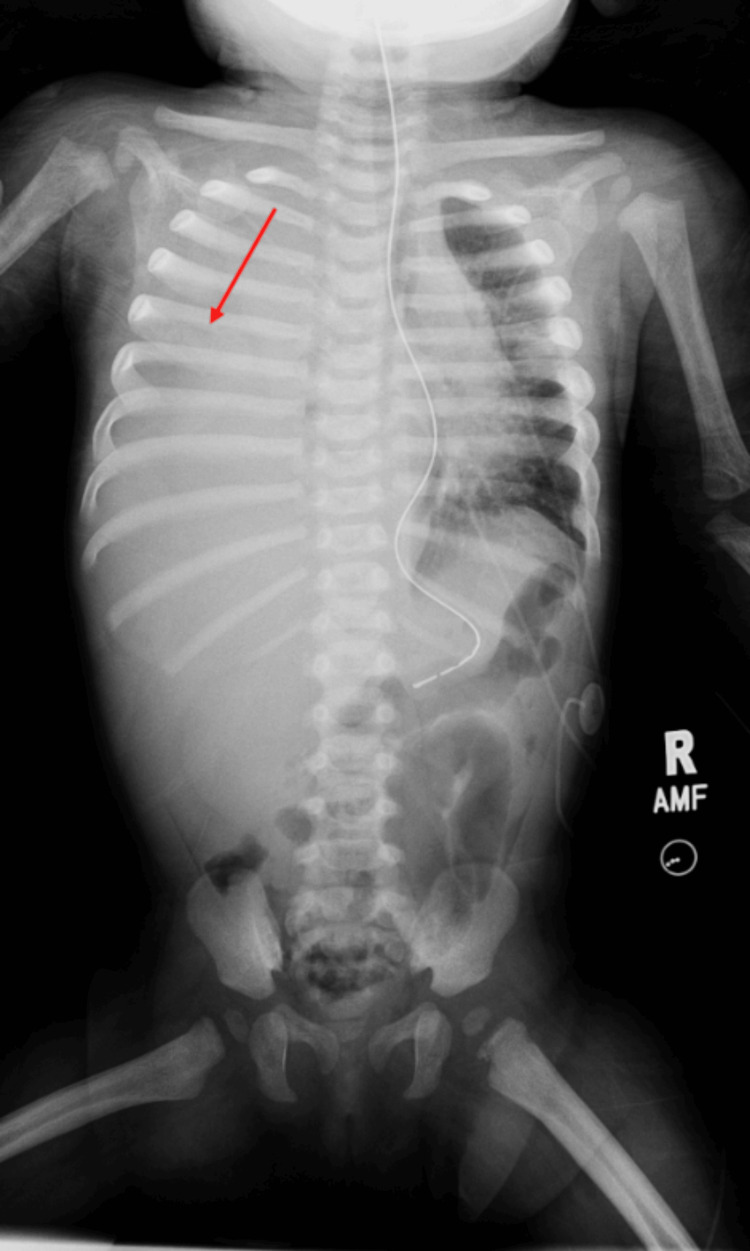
Chest abdomen radiograph showing near-complete opacification of the right hemithorax concerning for a large pleural effusion in addition to a periosteal reaction of the left ribs and the proximal right humerus suspicious for subacute fractures and concerning for non-accidental trauma.
